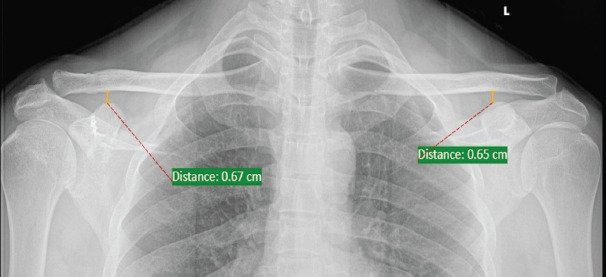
Stress radiograph after two years post-op showing ACJ articulation.
